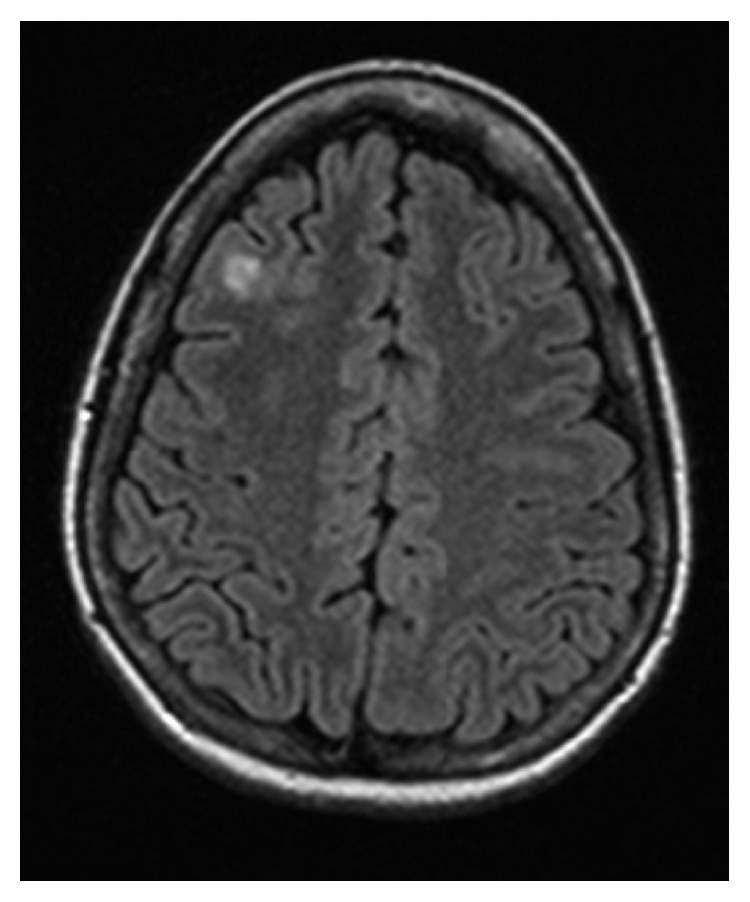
T2 FLAIR signal hyperintensity seen within the right middle frontal gyrus which did not enhance after contrast. There is no adjacent leptomeningeal/dural or parenchymal contrast enhancement.
